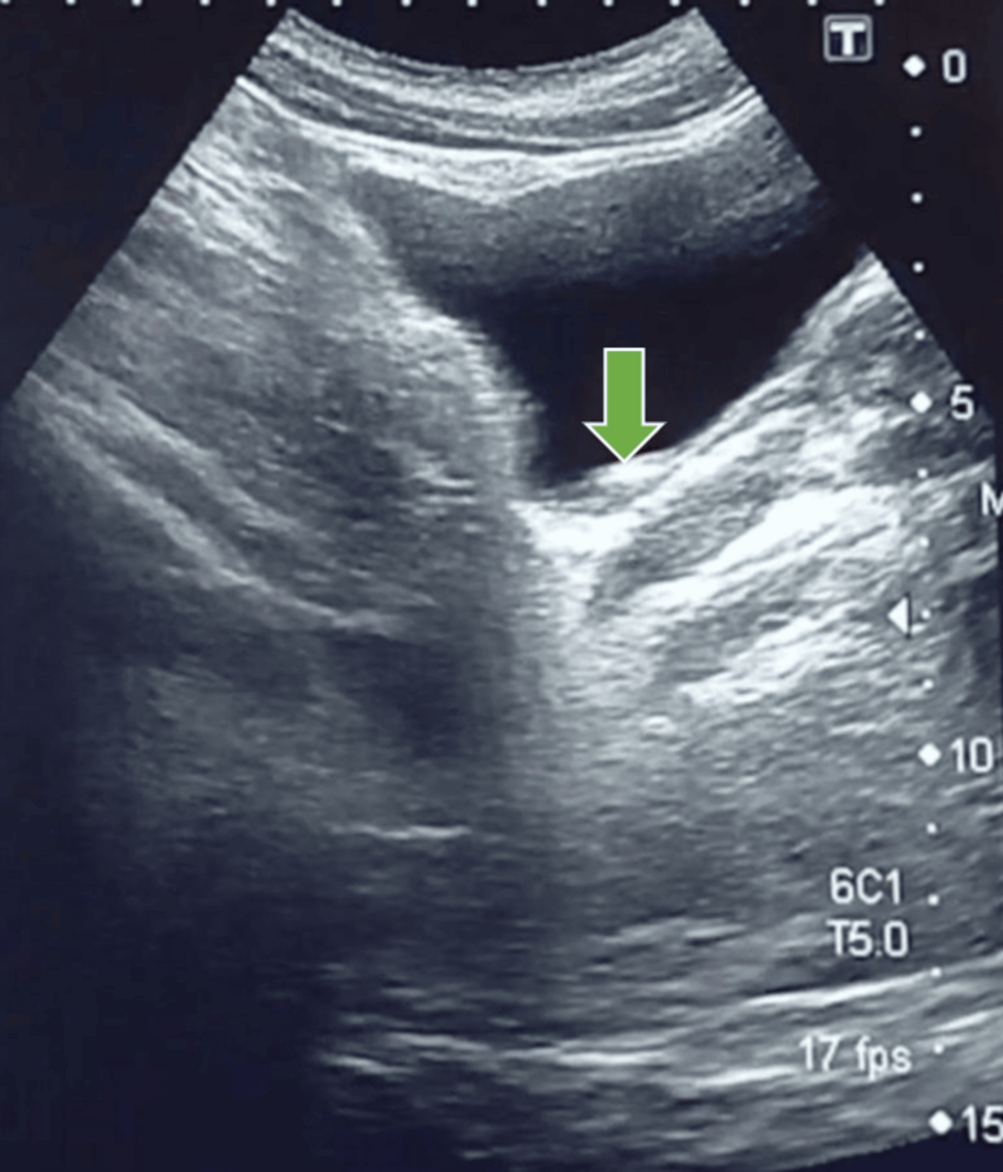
Ultrasound Demonstrating Distal Ureteric Calculus with Mild Upstream Dilatation Ultrasound of the pelvis demonstrating an echogenic focus with posterior acoustic shadowing at the right vesico-ureteric junction (green arrow), consistent with about 5 mm distal ureteric calculus causing mild upstream dilatation.
